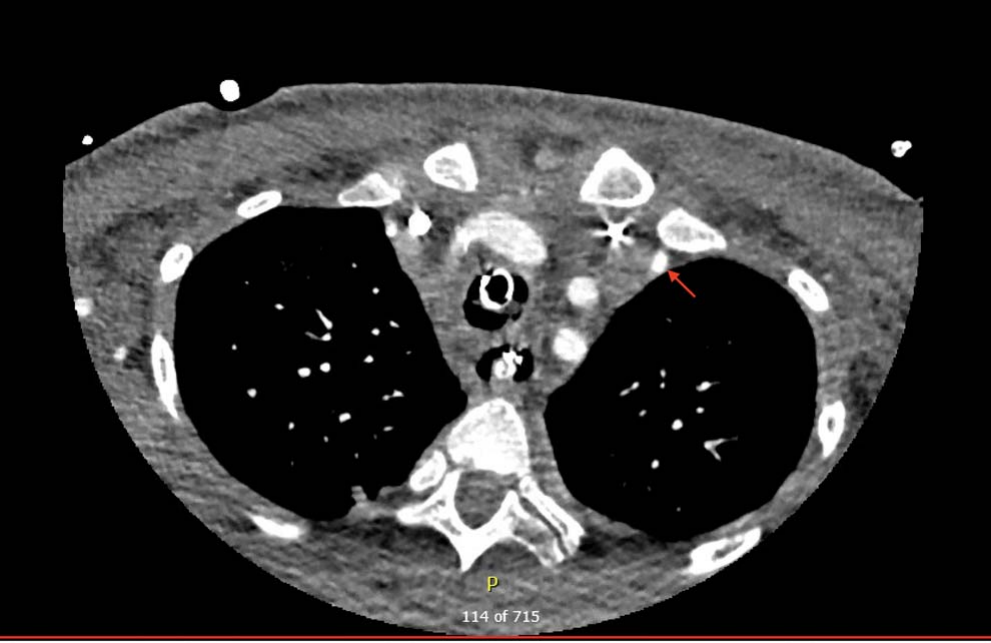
Computed tomography with contrast of the head and neck on day 2 (axial view) demonstrated dilated left internal mammary artery with possible bleeding (arrow).
